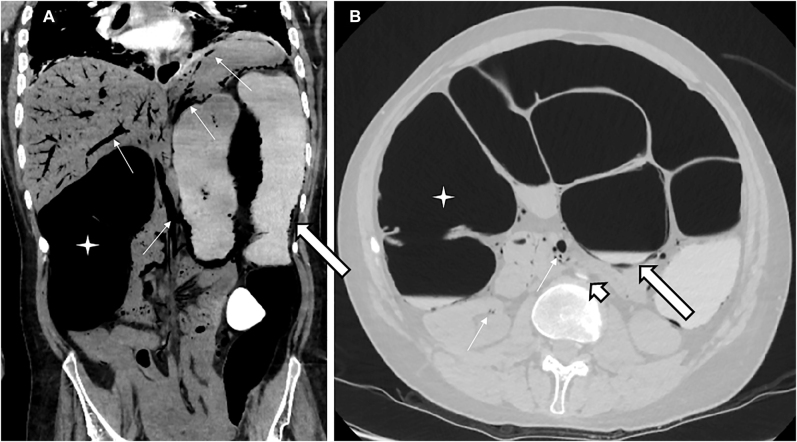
A CT scan of the abdomen showed massive dilatation of the colon (star), including pneumatosis coli (long bold arrows), extensive gas formation (thin arrows) within the mesenteric veins and arteries, including massive amounts of portal gas in the entire portal basin of the liver, splenic vein, renal veins, and the abdominal aorta, leading to disruption of perfusion (short bold arrow).
